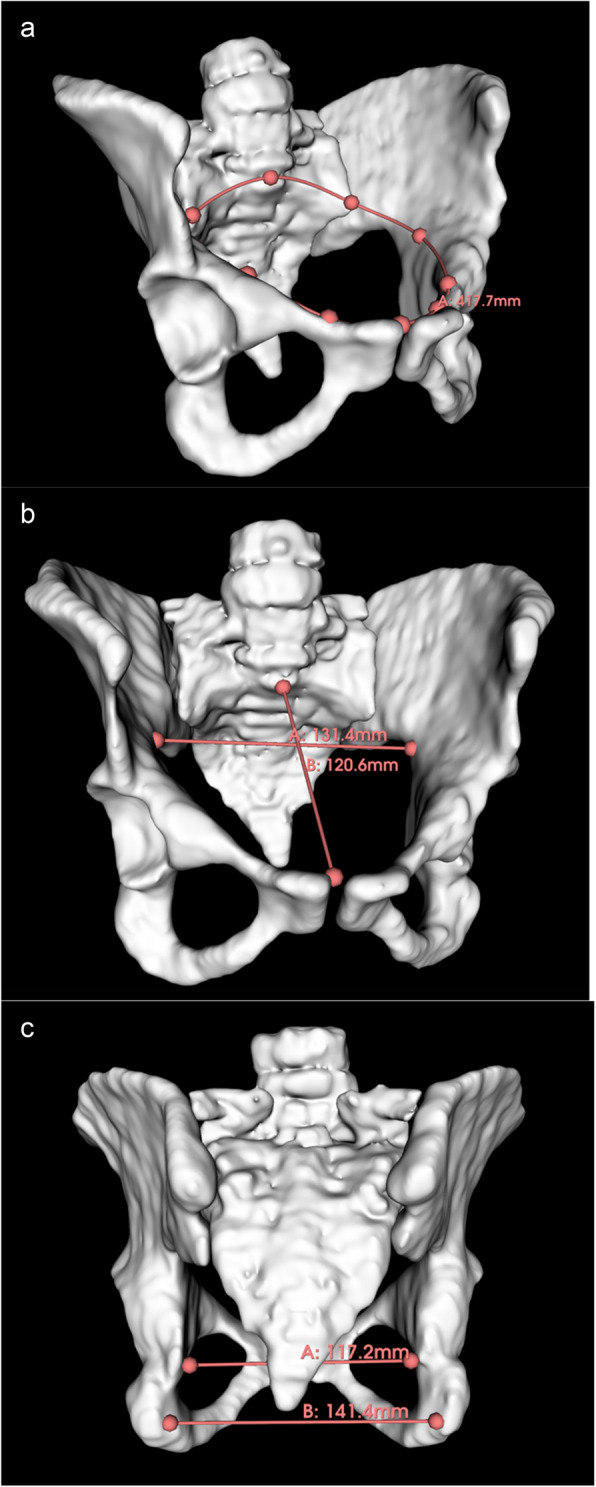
3D model of the fetal pelvis segmented from T2w MRI. (a) measured circumference of the inlet (b) transverse inlet (A), and the obstetric conjugate (B) (c) interspinous (A) and intertuberous distances (B).
